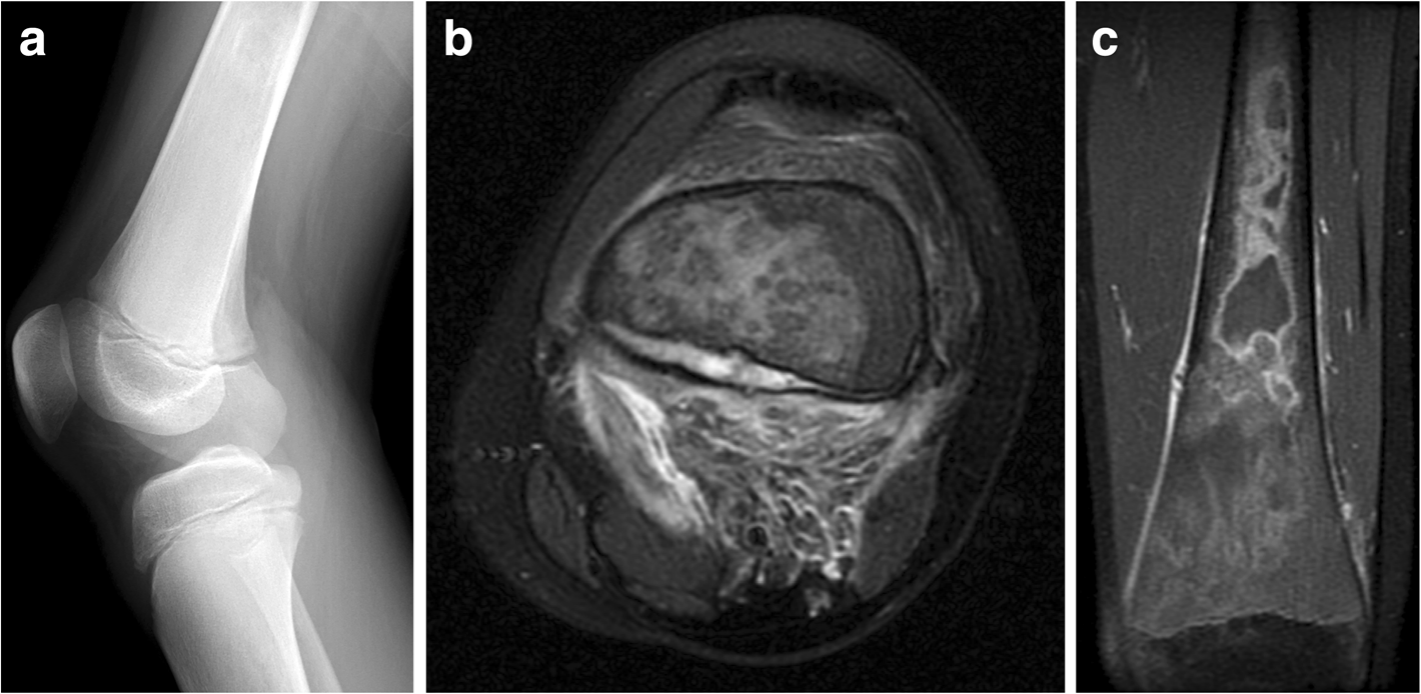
Bone infarct and subperiosteal hemorrhage mimicking osteomyelitis in Gaucher disease. A 13-year-old male presented with atraumatic knee pain and swelling. Initial lateral knee radiograph (a) showed subtle periosteal reaction along the posterior metadiaphysis of the distal femur and ill-defined intramedullary sclerosis within the femoral shaft. Follow-up MRI demonstrated intramedullary marrow edema and focal subperiosteal fluid with surrounding inflammatory changes in the popliteal fossa thought to be related to subperiosteal hemorrhage on axial T2-weighted image (b). Coronal post-contrast T1 fat-saturated image (c) demonstrated peripheral serpentine enhancement of areas of bone infarction within the distal femur. The patient was subsequently diagnosed with Gaucher disease after initial workup for hematopoietic malignancy
